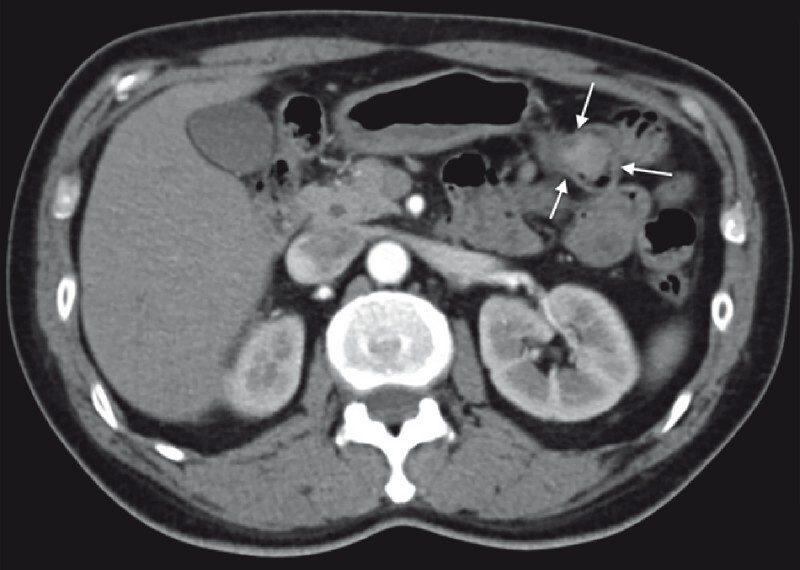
Computed tomography scan showing enhancement of the lesion (arrows).
